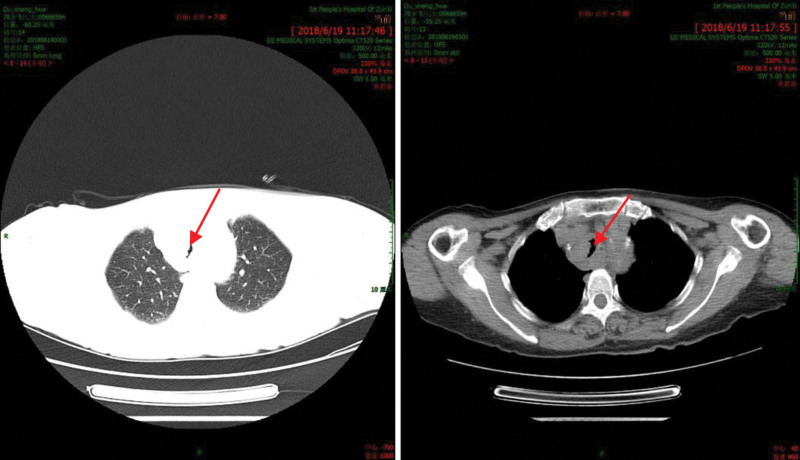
Computed tomography (CT) scan of the chest indicating soft-tissue lumen eminence and tracheal stenosis in the thoracic segment of the trachea.
